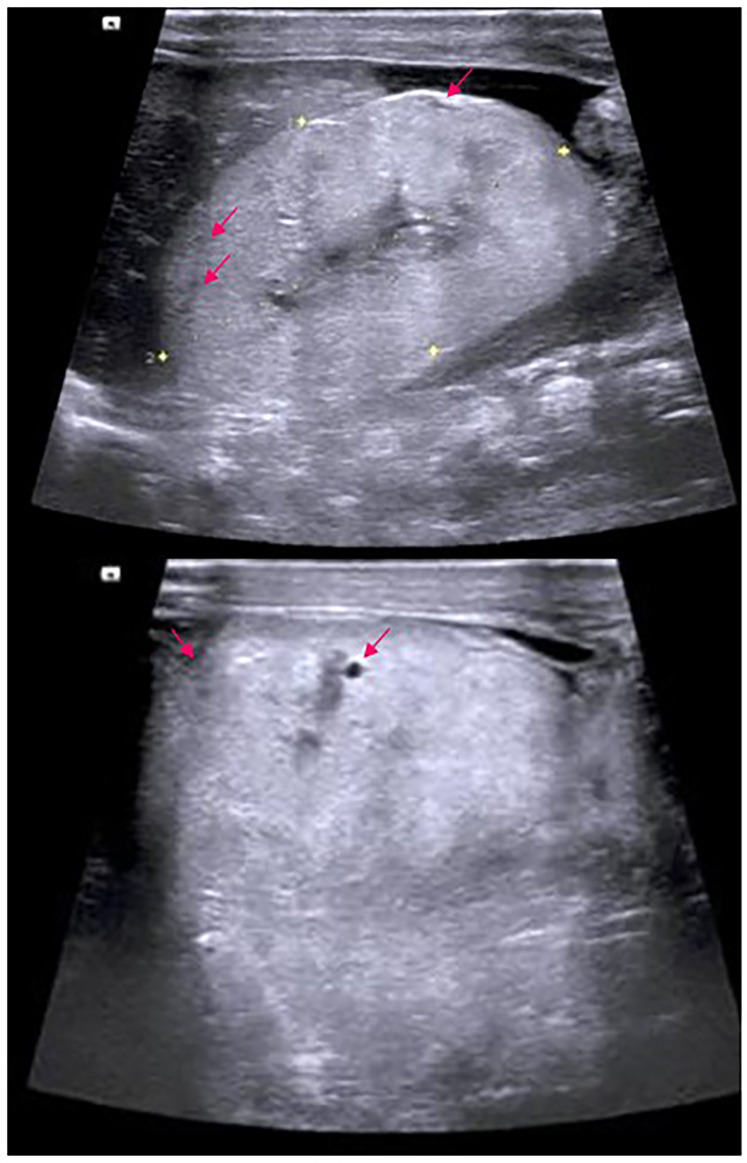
Renal ultrasonography in a child with WT1 p.Arg467GIn. Ultrasonography of the proband (II-3) at 22 days of age shows bilaterally enlarged kidneys with hyperechoic, thickened parenchyma (right kidney: 54 mm × 32 mm, top panel; left kidney: 53 mm × 36 mm, lower panel). The kidneys retain a grossly normal overall shape but showed poor corticomedullary differentiation. Multiple cysts of varying sizes (arrows) are scattered throughout the renal parenchyma.
